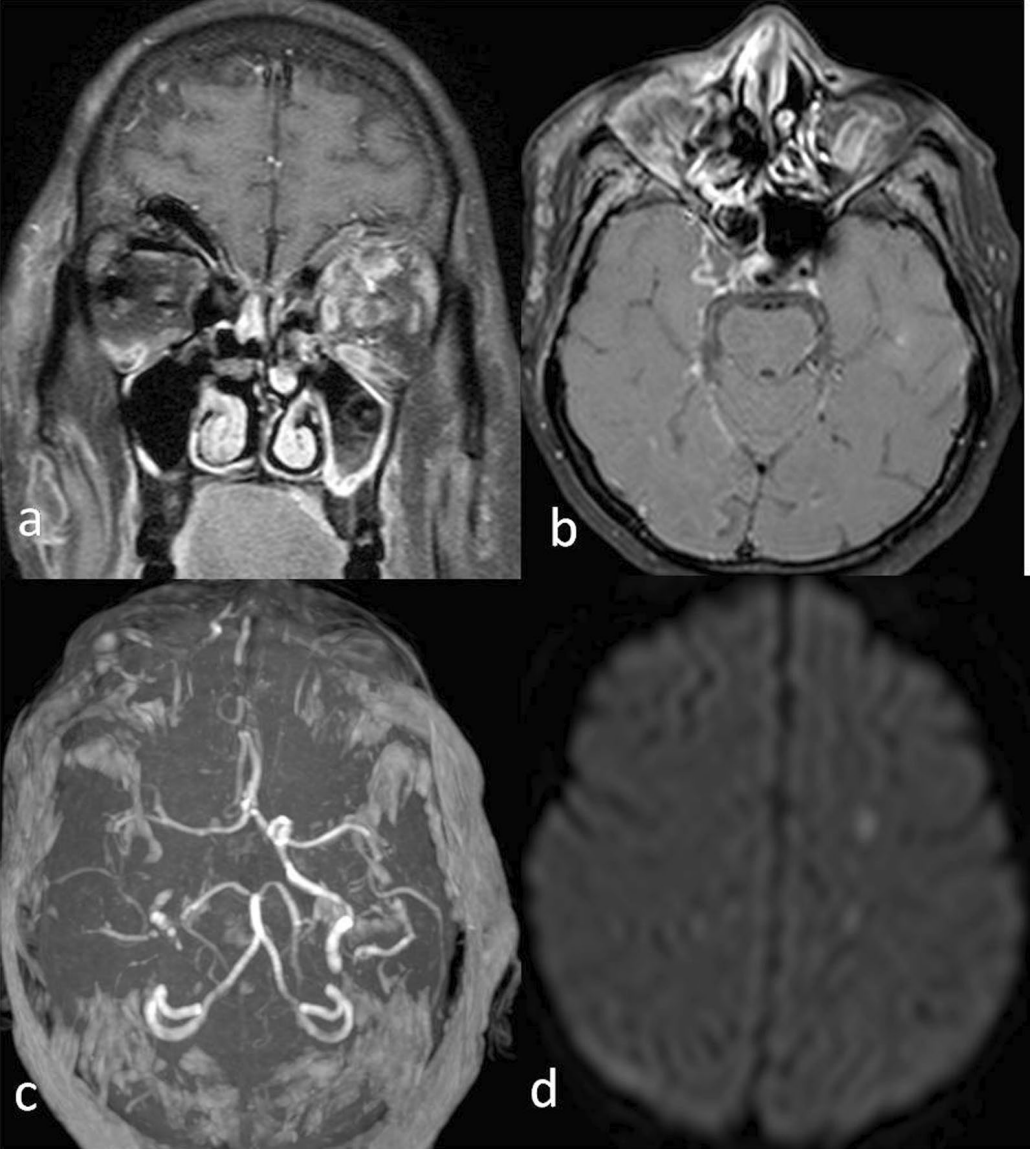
A 50-year-old diabetic patient with a history of COVID infection 1 month back, presented with acute onset nasal blockage and discharge, swelling on the right side of the face. Post-contrast T1 W coronal (a) and axial (b) images showing mucosal thickening of bilateral maxillary sinuses with contents in left maxillary sinus with the black turbinate sign of right middle turbinate. B/l Intraorbital extension of infection seen with left optic neuritis. Right cavernous sinus invasion is seen with loss of signal void of right intracavernous ICA. MIP images of MRA (c) show right ICA thrombosis. (d) DWI image showing few septic infarcts in left centrum semiovale. Findings were consistent with Invasive mucormycosis
